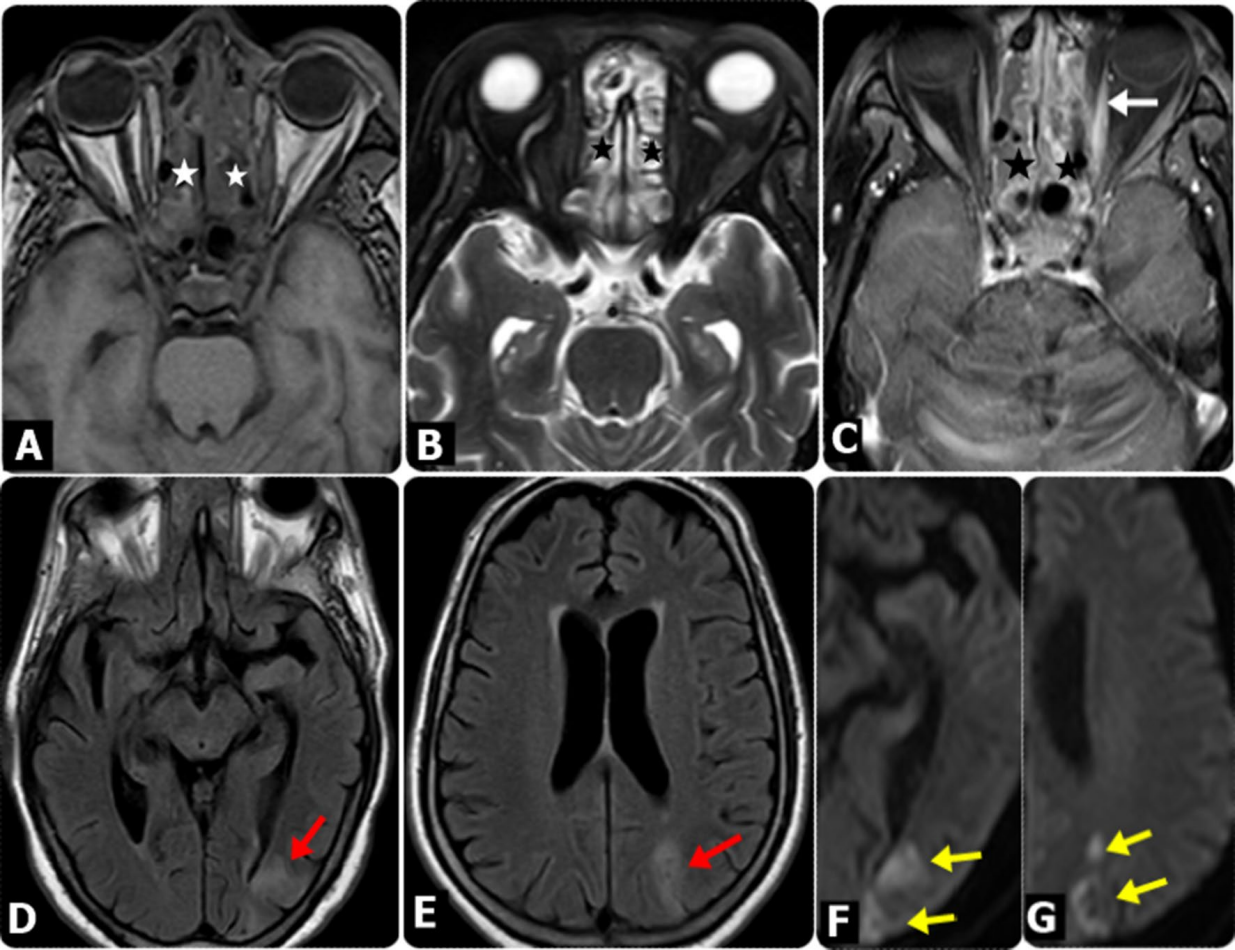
MRI findings in diabetic male patient, 55 years old, presented mainly with Lt. periocular and eyelids edema, following COVID-19. (A) Axial T1W, (B) and Axial T2 SPIR images showed bilateral ethmoidal sinusitis (Asterisks) and mild thickening of Lt. medial rectus muscle. C Axial post-contrast fat-saturated T1WI showed bilateral ethmoidal sinusitis with heterogeneous mucosal enhancement with mild thickened and enhanced Lt. medial rectus muscle (white arrows). D and E Axial FLAIR images; showed patchy hyperintense areas representing embolic acute infarcts at the Lt. occipital & posterior parietal regions (red arrows) which presented restricted diffusion on DW images (yellow arrows) at b-value 1000 (F) and (G)
